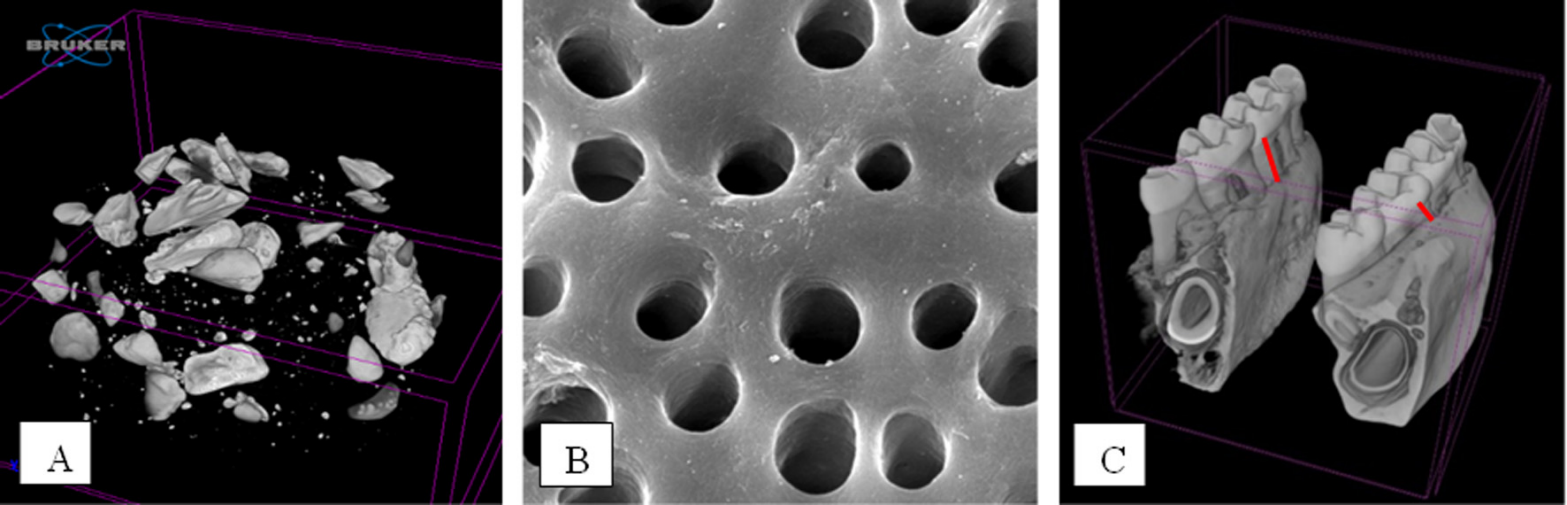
(A) Overview of the DDM scaffold particles (μ-CT photographs) (B) SEM photographs of DDM Scaffold visible pores (5000 × Magnification) (C) Overview of the periodontitis model shows alveolar bone resorption (red arrows indicate alveolar bone resorption (μ-CT photographs).
